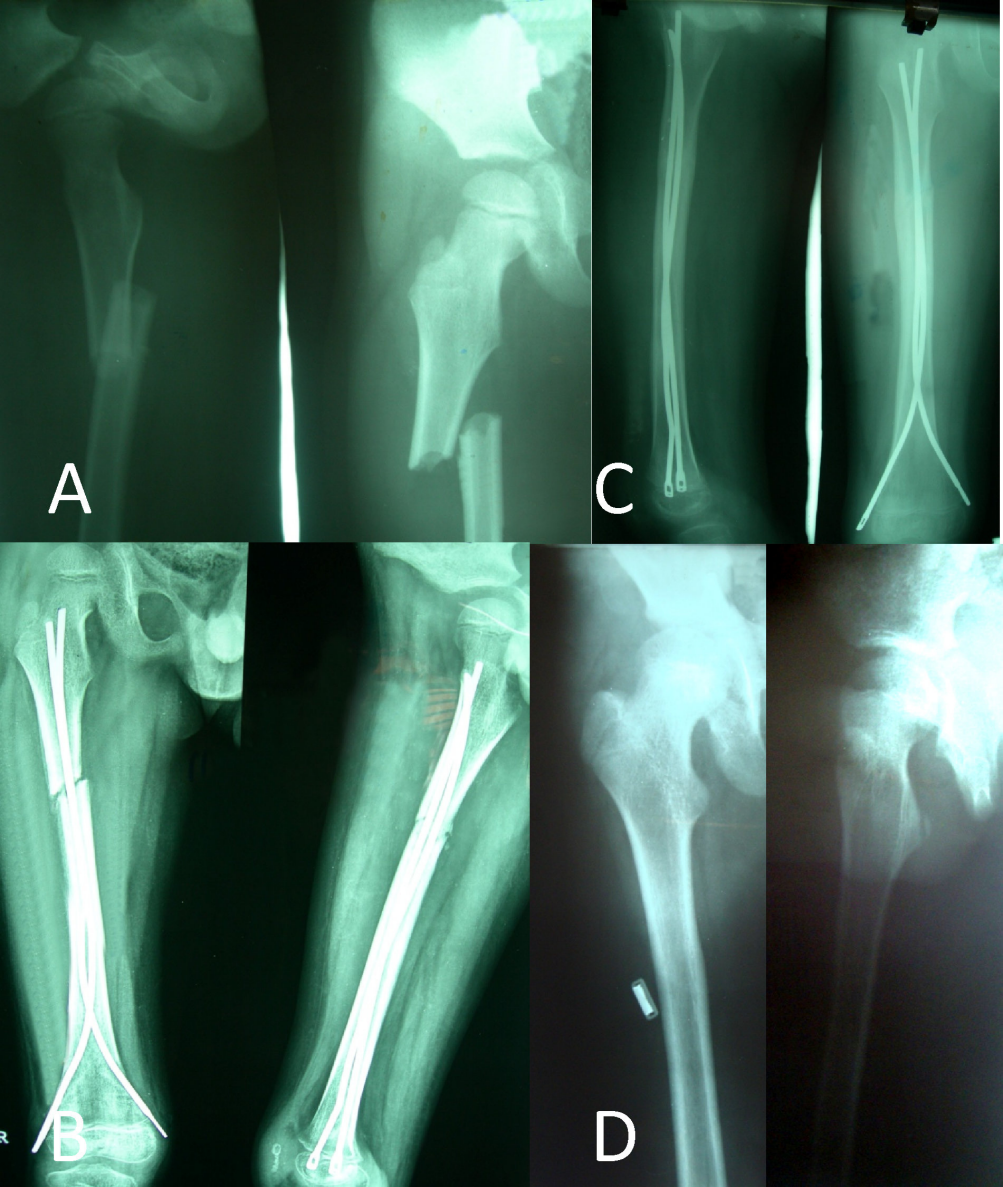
Femoral shaft fracture of right side in a 6 year old child treated with Ender's nail. Radiographs revealed displaced femoral shaft fracture (A) of right side. Excellent fracture reduction (B) was achieved which was maintained till fracture union (C) and final follow up radiographs at 6 years postoperatively (D) demonstrated neutral alignment in both anteroposterior and lateral views
